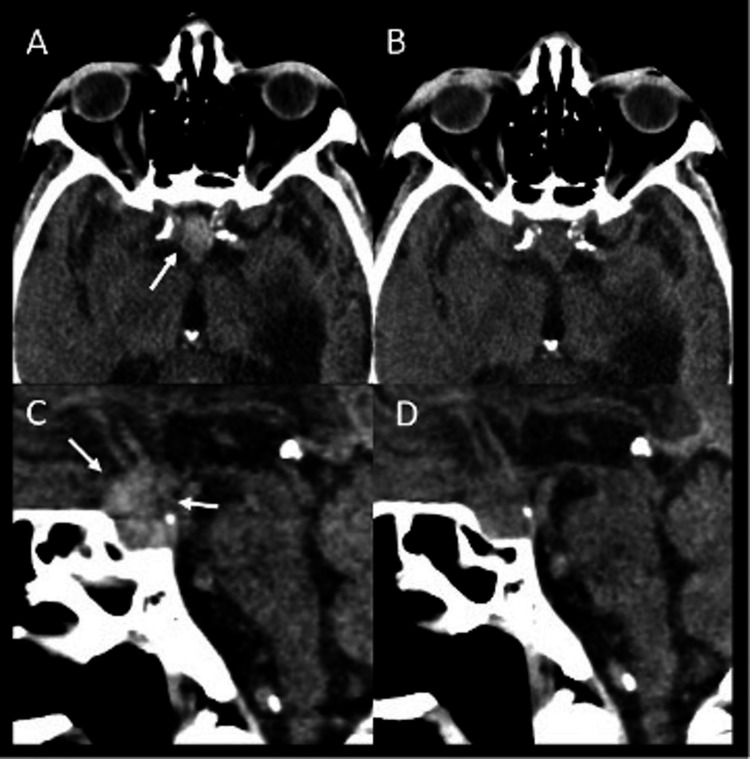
Unenhanced CT images in axial (A,B) and sagittal (C,D) plane demonstrates pituitary adenoma with interval development of hyperdensity within the lesion (arrows in A,C) compared to prior CT 2 weeks prior (B,D) compatible with interval pituitary hemorrhage.
